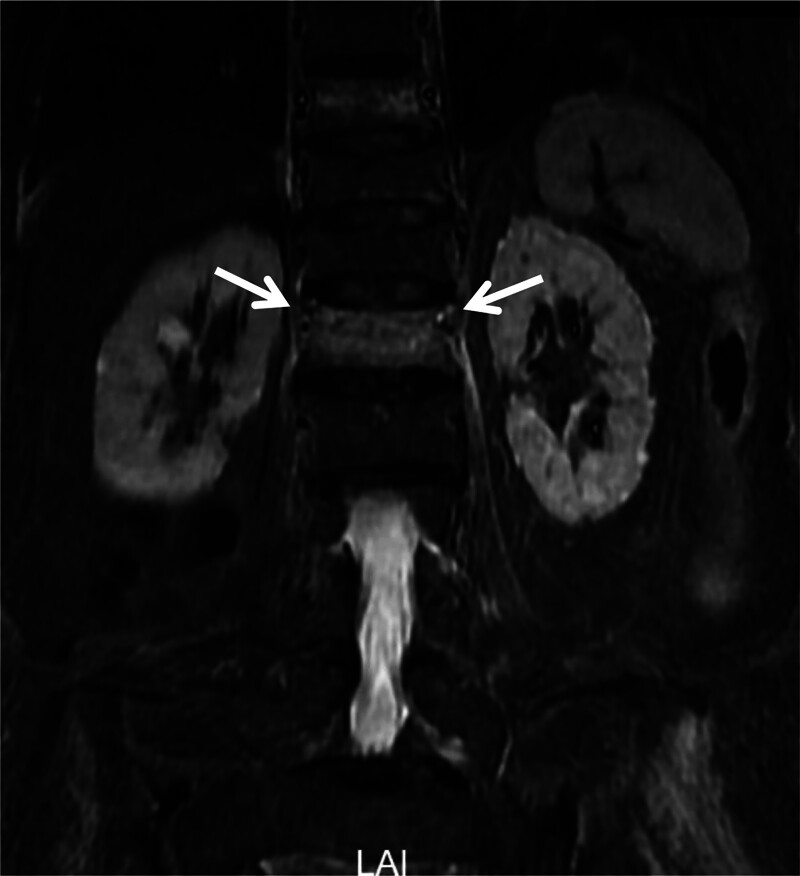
The arrow points to the L1 segmental artery, which is displaced cranially into the upper quarter of the vertebral body on this coronal MRI—a region contrary to the traditional “safe window” of the posterosuperior third.
